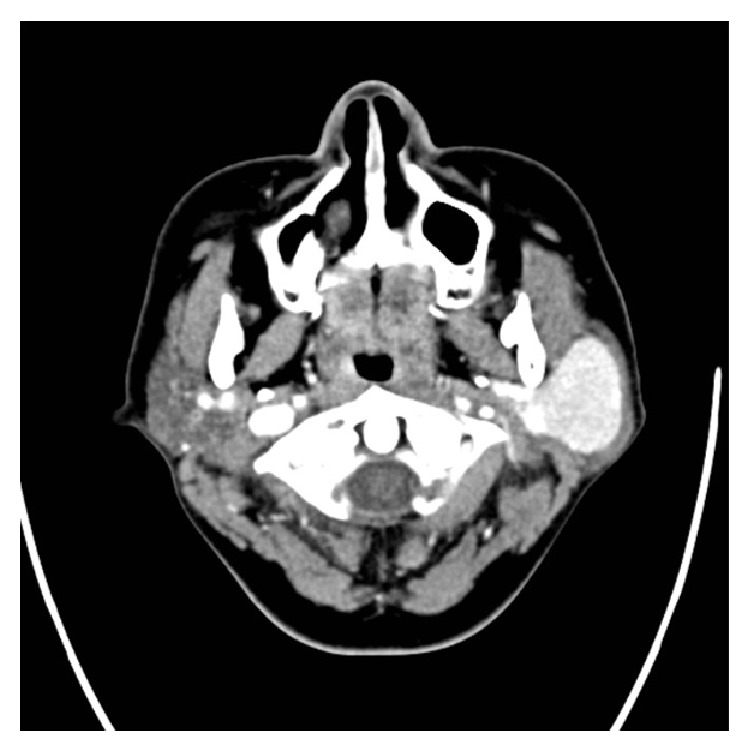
CT head and neck axial cut, after contrast, soft tissue window: a well-defined, oval shaped solid lesion involving the left superficial lobe of the parotid gland. The lesion shows a diffuse intense enhancement after IV contrast media injection.
